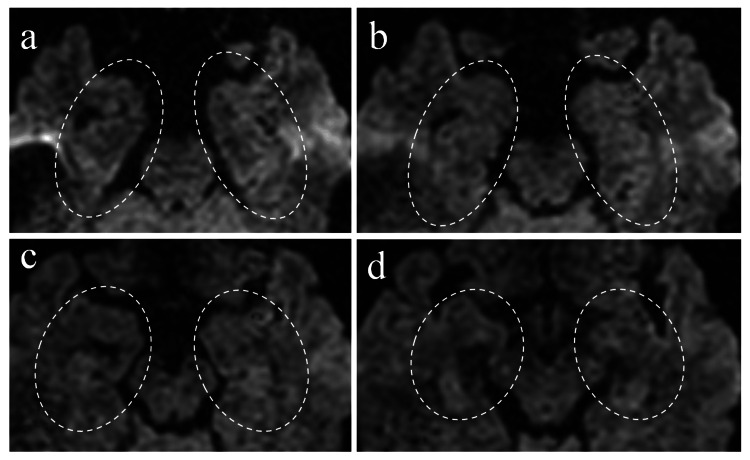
MRI imaging with 2 mm-thickness thin-slice three days after amnestic episode showing the absence of hippocampal lesions No diffusion restriction area was detected in the hippocampal areas (white ellipses) even with the careful investigation utilizing a thin-slice MRI three days after the amnestic episode, making the diagnosis of TGA less likely. Axial diffusion-weighted imaging with TR/TE = 5000/84.3 ms MRI: magnetic resonance imaging; TGA: transient global amnesia
